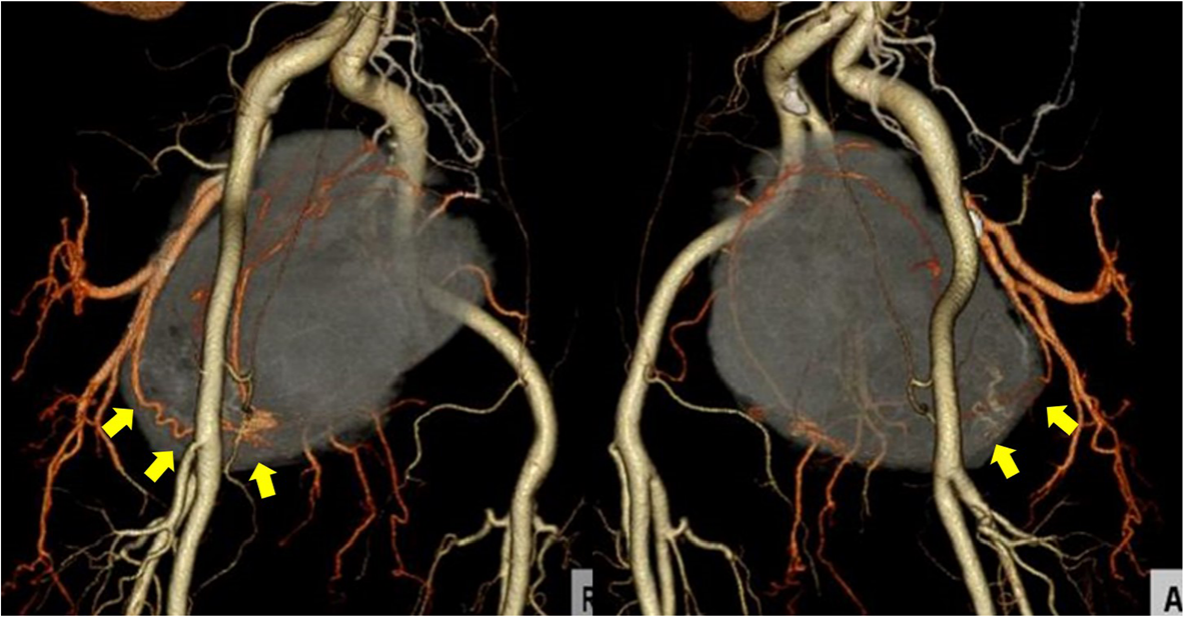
CT-angiography image showing the feeding vessels of the tumor branching from the right and left internal iliac arteries (arrow)
